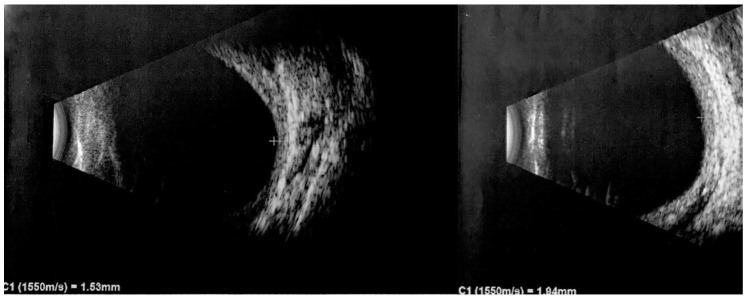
B-scan ultrasound examination. Diffuse choroidal hemangioma in both eyes, especially in the left eye (LE). Choroidal thickness of 1.53 mm and 1.94 mm in the right eye (RE) and LE, respectively. From [6].
